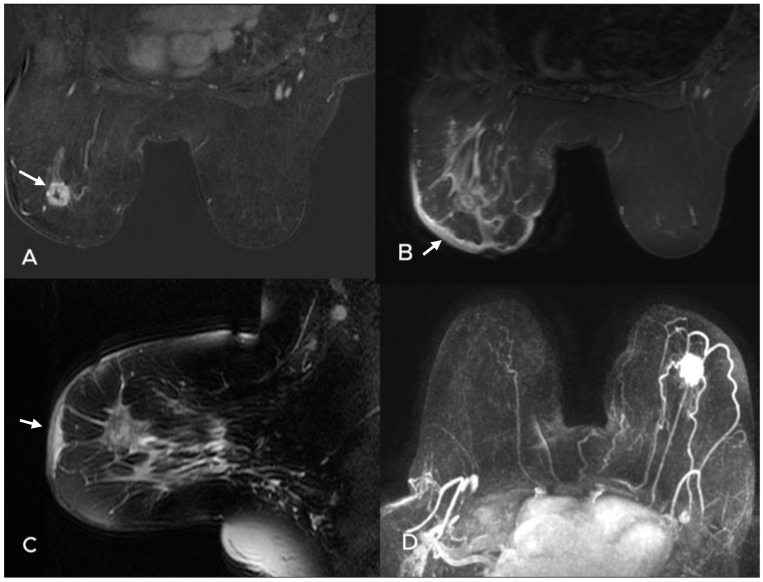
A 51-year-old woman with redness and thickening of the skin in the left breast. Preoperative MRI examination revealed a 2.5 cm round mass with irregular margins in the upper outer quadrant of the left breast. It demonstrates heterogeneous enhancement on axial substracted image (A), and subcutaneous edema on axial and sagittal STIR TSE images (B,C) (white arrows). A round-shaped lymph node is also seen in the axilla on the sagittal T2W (C) and axial maximum intensity projection images (D). Pathology: IDC, ER: Low positive 1–10%, PR: Negative, HER2: Score 3/++++, Ki67: 55% Core needle biopsy of the lymph node: metastasis.
